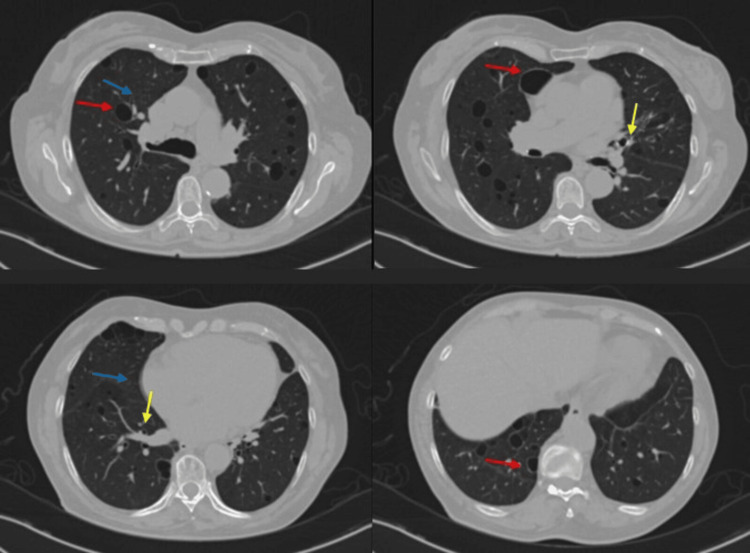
Chest CT scan obtained during follow-up in 2024: pulmonary axial window. Radiological improvement of the ground-glass infiltrates (blue arrow) and the slight thickening of the bronchovascular interstitium (yellow arrow) can be seen. There are no changes in the thin-walled cysts with a predominance in the medial and inferior lung (red arrow).
